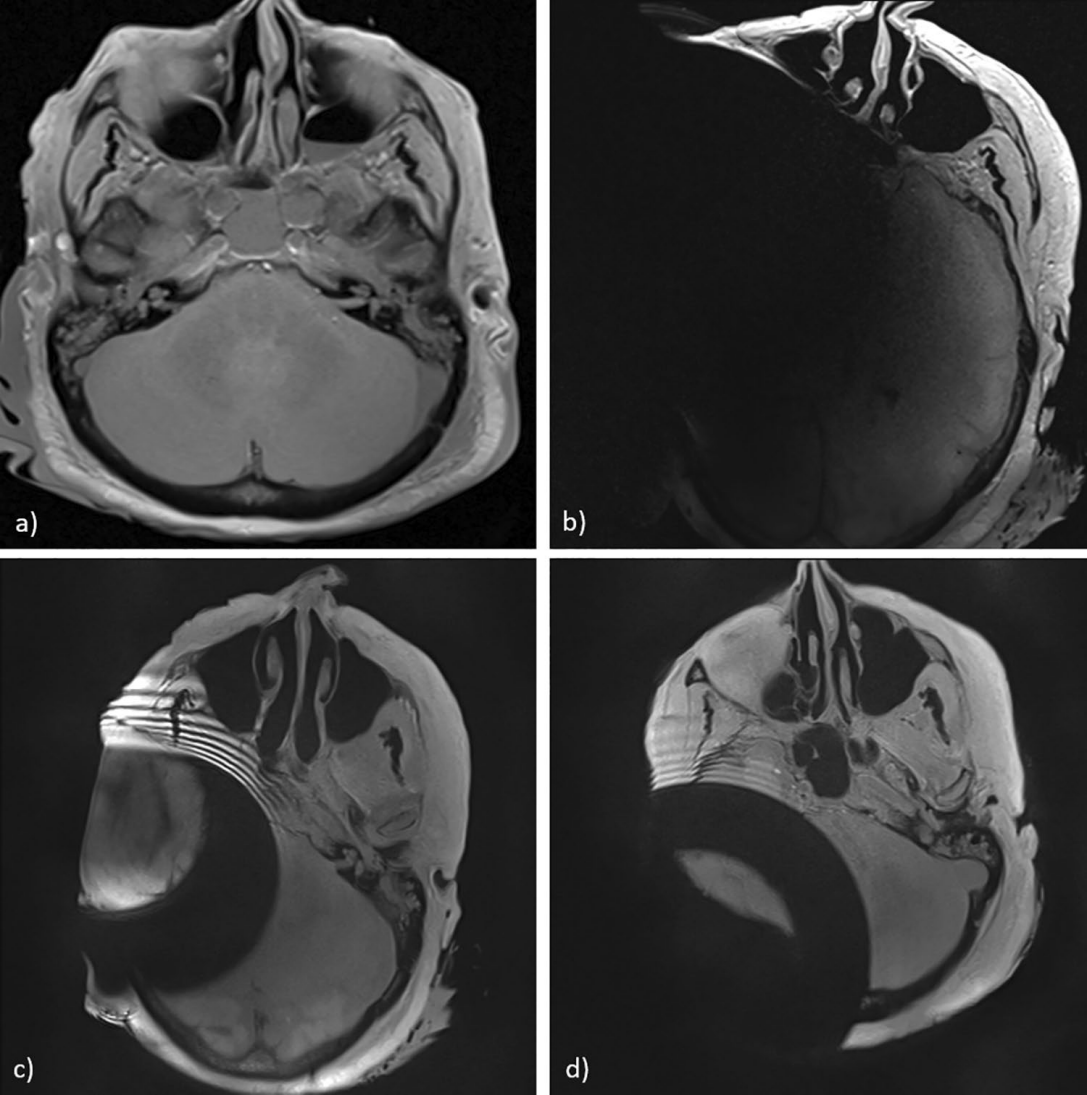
MR images from one of the two head samples. All MR images shown here are taken from an axial T1 sequence. a Pre-implantation scan used as a reference. b Post-implantation scan without metal artifact reduction sequences. c Post-implantation scan with SEMAC-VAT WARP artifact reduction sequence: implant placed on the mastoid. d Post-implantation scan with SEMAC-VAT WARP artifact reduction sequence: implant placed in retrosigmoid location
